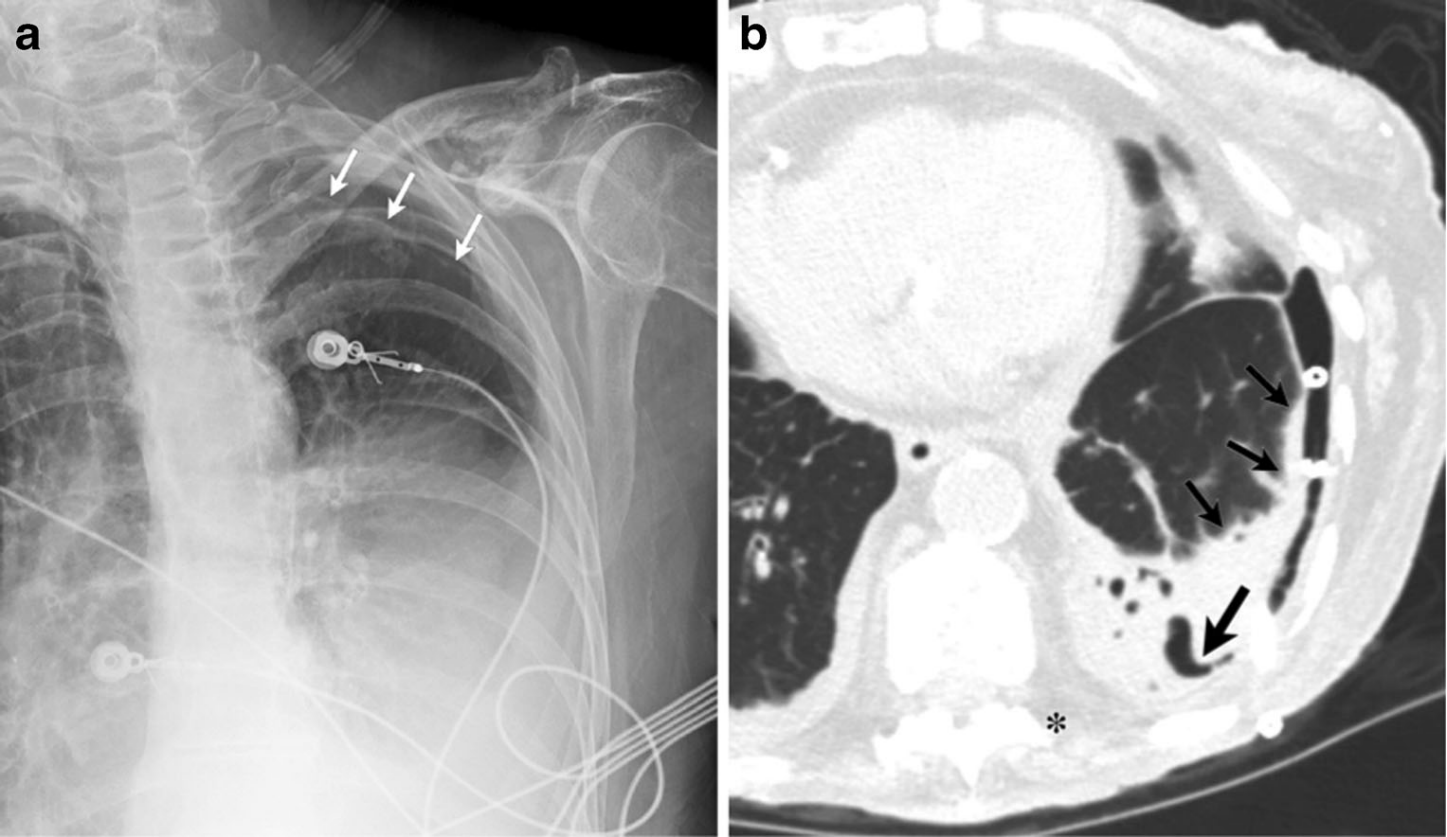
A 70-year-old female with a history of left-sided empyema, status post drainage. Frontal chest radiograph (a) demonstrates a small left apical pneumothorax (*white arrows*). Axial CT (b) demonstrates a broncho-pleural fistula (*thicker black arrow*) secondary to parenchymal necrosis. In addition, pleural thickening (fibrothorax) surrounding the left lower lobe (*small arrows*) “traps” the lung, preventing it from fully expanding. This is also an example of an ex vacuo pneumothorax
